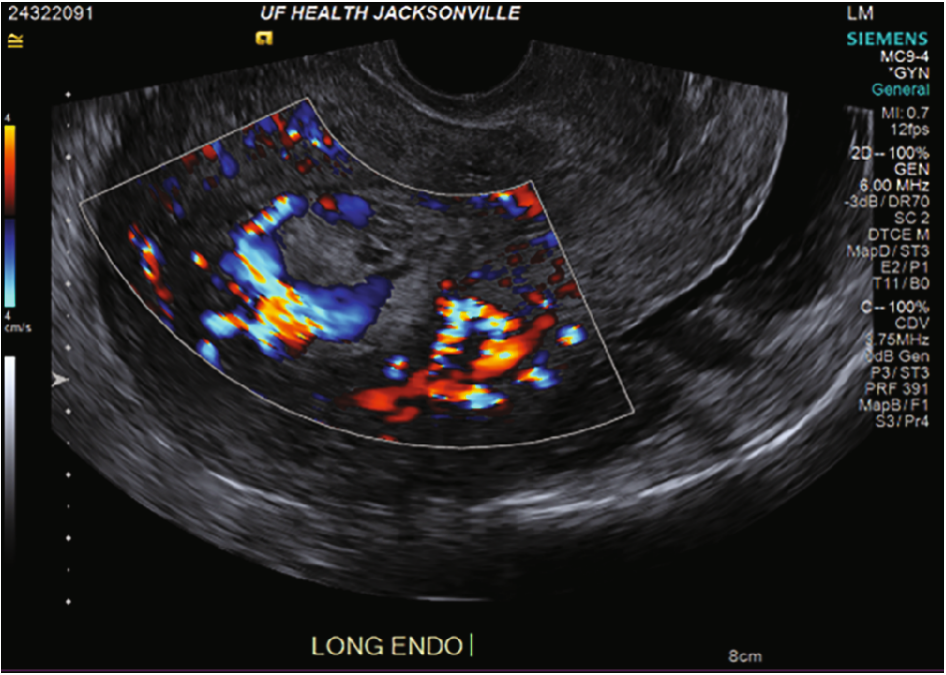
Transvaginal ultrasound demonstrating a thickened and heterogenous endometrium measuring 2.7 cm with hypervascularity.
